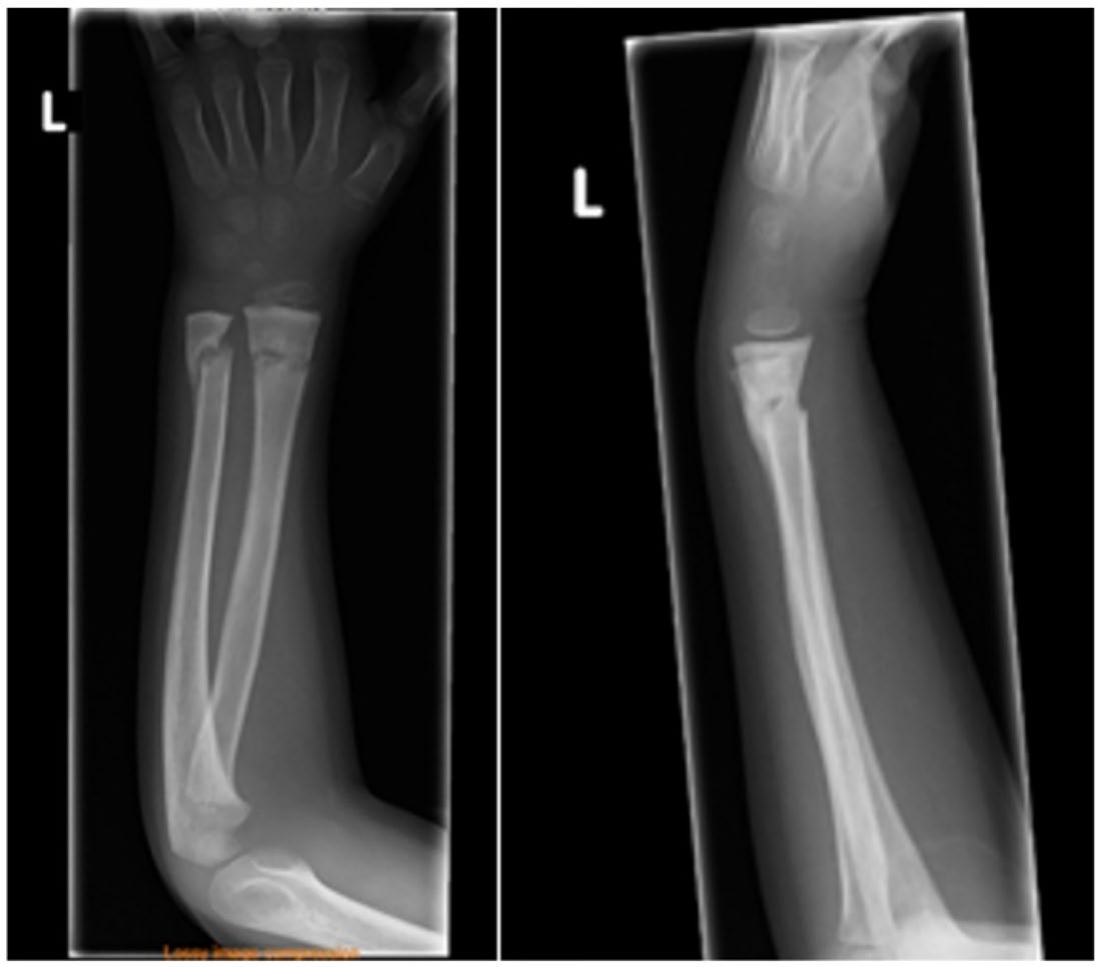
X-ray follow-up 4 weeks after trauma.
